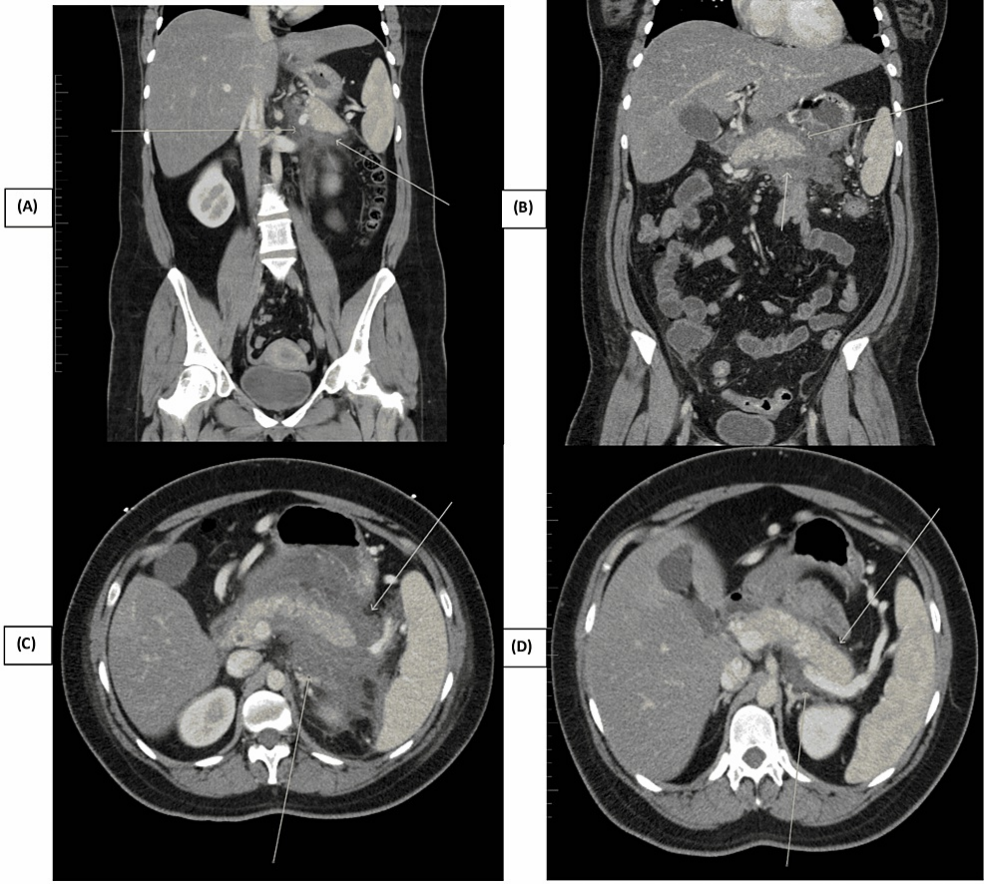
CT abdomen and pelvis showing acute pancreatitis with hepatic steatosis (A-D)
